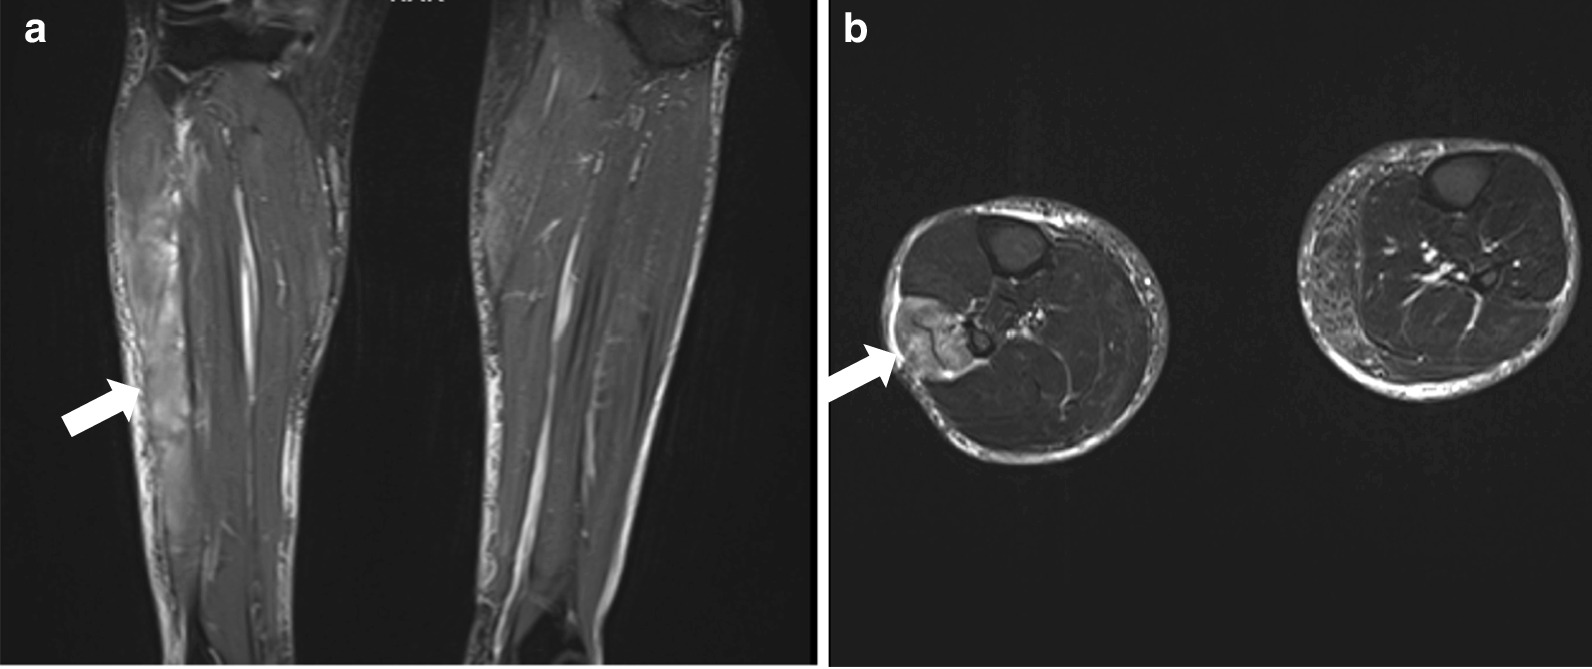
Contrast-enhanced magnetic resonance imaging of both lower legs: a coronal cut and b axial cuts. Both demonstrate (indicated by a white arrow) right-sided gross edema and swelling of the lateral lower leg compartment involving the peroneus brevis and longus muscles, consistent with acute lateral compartment syndrome. No intramuscular hemorrhage is evident. There is extensive soft tissue edema. Normal appearance of the anterior, deep and superficial compartments. Diagnosis favors acute lateral compartment syndrome of the right leg
